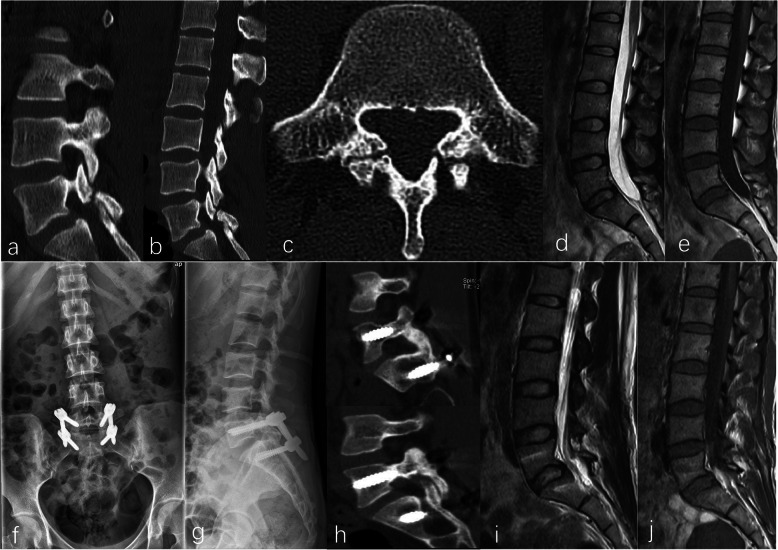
A 23-year-old woman was admitted to our institution due to chronic low back pain without lower extremity pain and numbness for 8 months. Bilateral pars defects at L5 was revealed on preoperative CT scans (a, b, c). Grade I disc degeneration at L5/S1 was seen on preoperative MRI images (d, e). The patient underwent single-level endoscopic-assisted direct repair of pars defect supplemented with percutaneous pedicle screw fixation successfully (f, g). Sagittal CT images at 11 months postoperatively revealed solid fusion at both sides (h). Obvious disc degeneration was not observed on MRI images (i, j)
